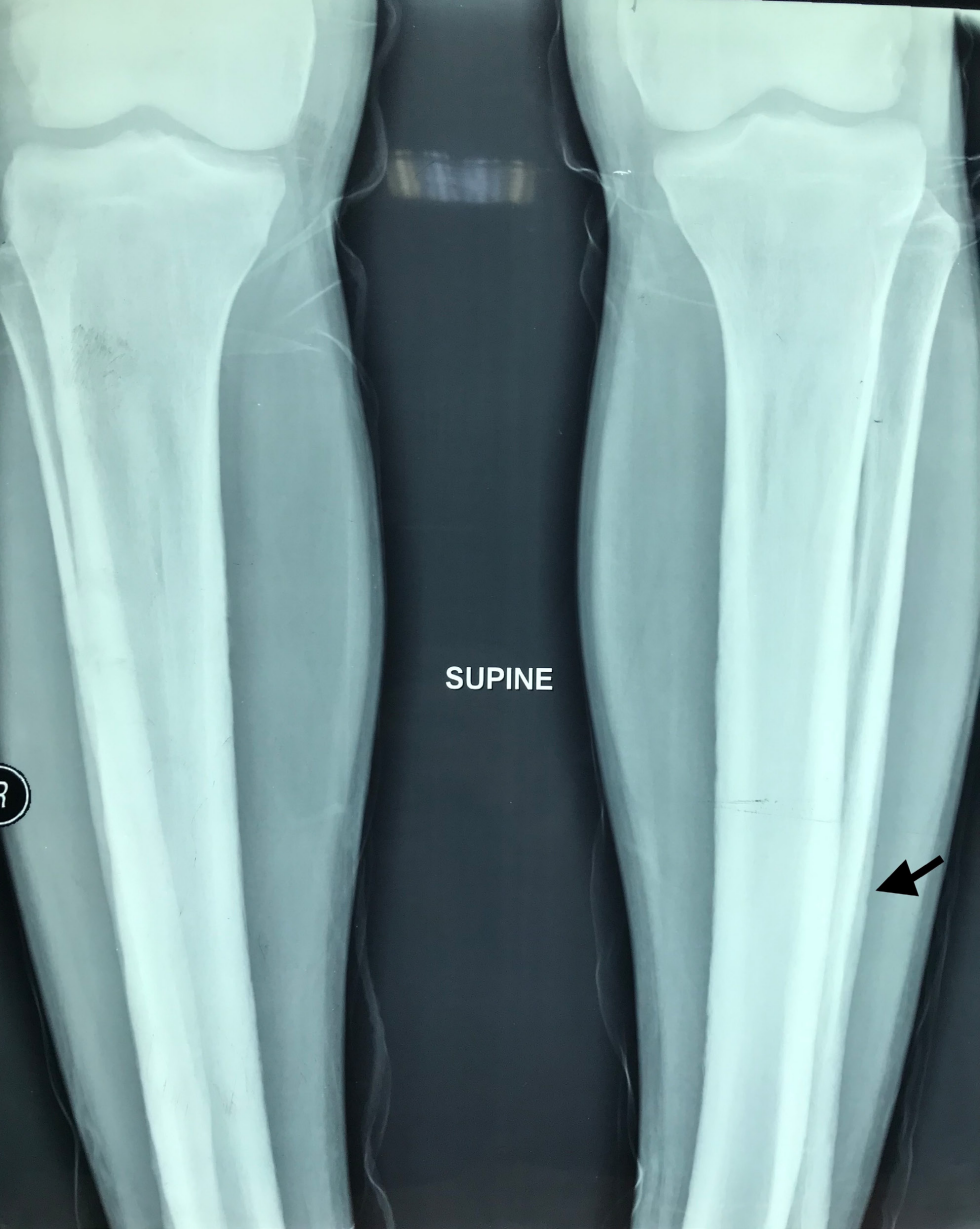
A radiograph revealed cortical thickening of the tibia and fibula, with periosteal bone deposition more apparent on the left fibula (arrow).
